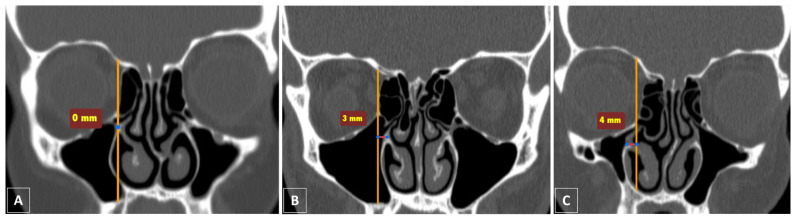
Classification of variations in the medial wall of the maxillary sinus relative to the medial orbital wall, as observed in coronal CT scan images of the paranasal sinuses. (A) Class I: the attachment site of the inferior turbinate is positioned in the same vertical plane as the line that crosses the IOS. (B) Class II: the attachment site of the inferior turbinate is positioned 3 mm medially to the vertical line. (C) Class III: the attachment site of the inferior turbinate is positioned 4 mm laterally to the vertical line.
